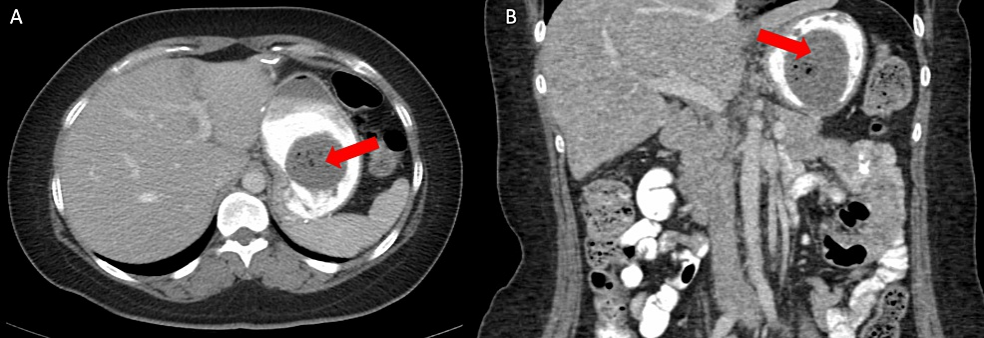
Computed tomography images of gastric bezoar. Computed tomography scan with oral contrast demonstrating gastric bezoar in axial (A) and coronal (B) views (red arrows).
